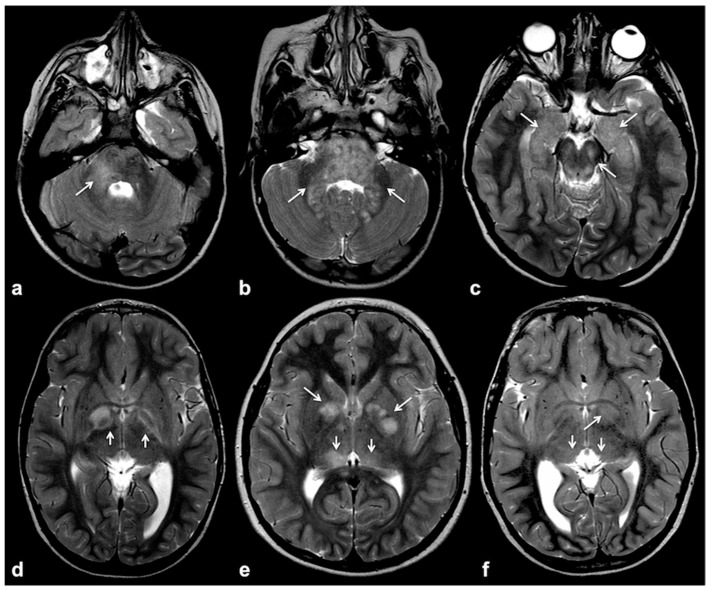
Typical focal abnormal signal intensity (FASI) localization to cerebellar pedicles and cerebellum (a,b), brainstem, cerebral peduncles and hippocampi (b,c), as well as basal ganglia (d–f) on axial TSE T2w MR images (white arrows).
